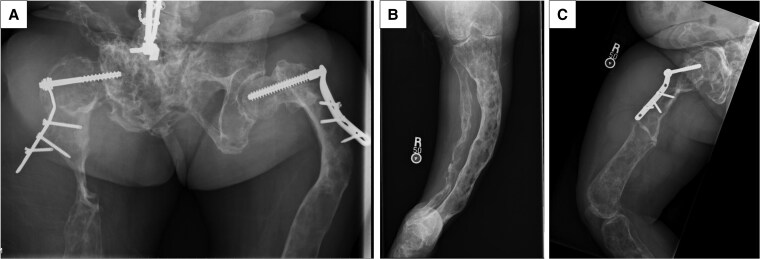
Plain radiographs of (A) the hips, (B) the right tibia and fibula, and (C) the right femur in a patient with McCune-Albright syndrome and polyostotic fibrous dysplasia. The images demonstrate extensive mosaic, ground-glass, and lytic changes involving all the visualized osseous structures, consistent with fibrous dysplasia. There is an increased risk of pathologic fracture, especially of the right femur and tibia-fibula noting cortical destruction with minimal residual bone mass. Bilateral proximal femoral fixation hardware is present with radiographic features suspicious for hardware loosening.
